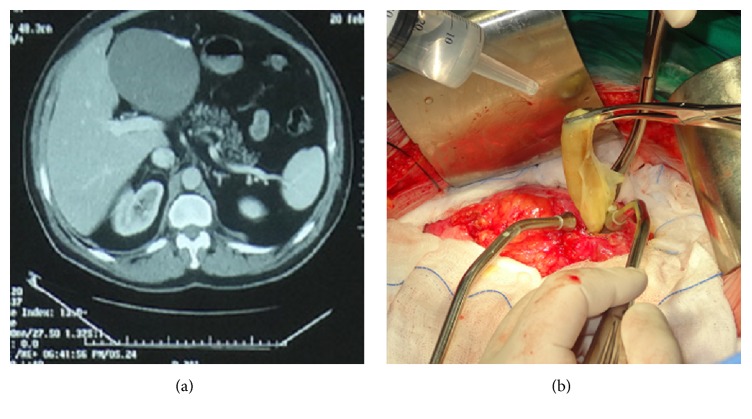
(a) Preoperative CT of a patient with a large left lobe hydatid cyst, obliterating the LPV and in contact with the RPV. (b) Intraoperative photo of the endocyst removal, after aspirating and opening the cyst.
